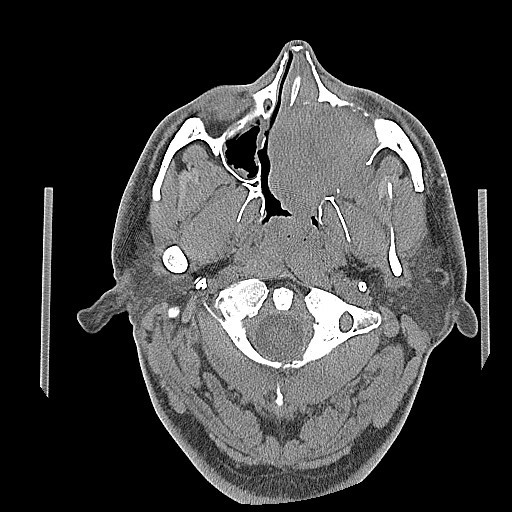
CT image of the brain, axial view – There is a large expansile soft tissue mass centered within the left maxillary sinus with extensive osseous dehiscence. Tumor extends into the left orbital floor, nasal cavity, left nasopharynx, left pterygopalatine fossa, left premaxillary space, and left infratemporal fossa.
